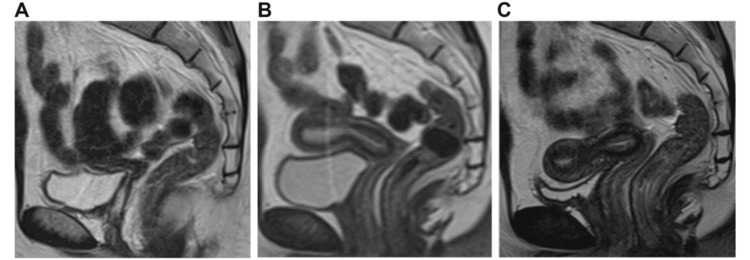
Changes in the size of the uterus. T2-weighted pelvic MRI shows uterine changes by sagittal section. The left figure represents the uterus at age 27 (initial visit), measuring 33 mm × 7 mm × 10 mm (A). The middle figure represents the uterus at age 28 (after one year of treatment), measuring 60 mm × 28 mm × 22 mm (B). The right figure represents the uterus at age 29 (after two years of treatment), measuring 70 mm × 28 mm × 23 mm (C).
